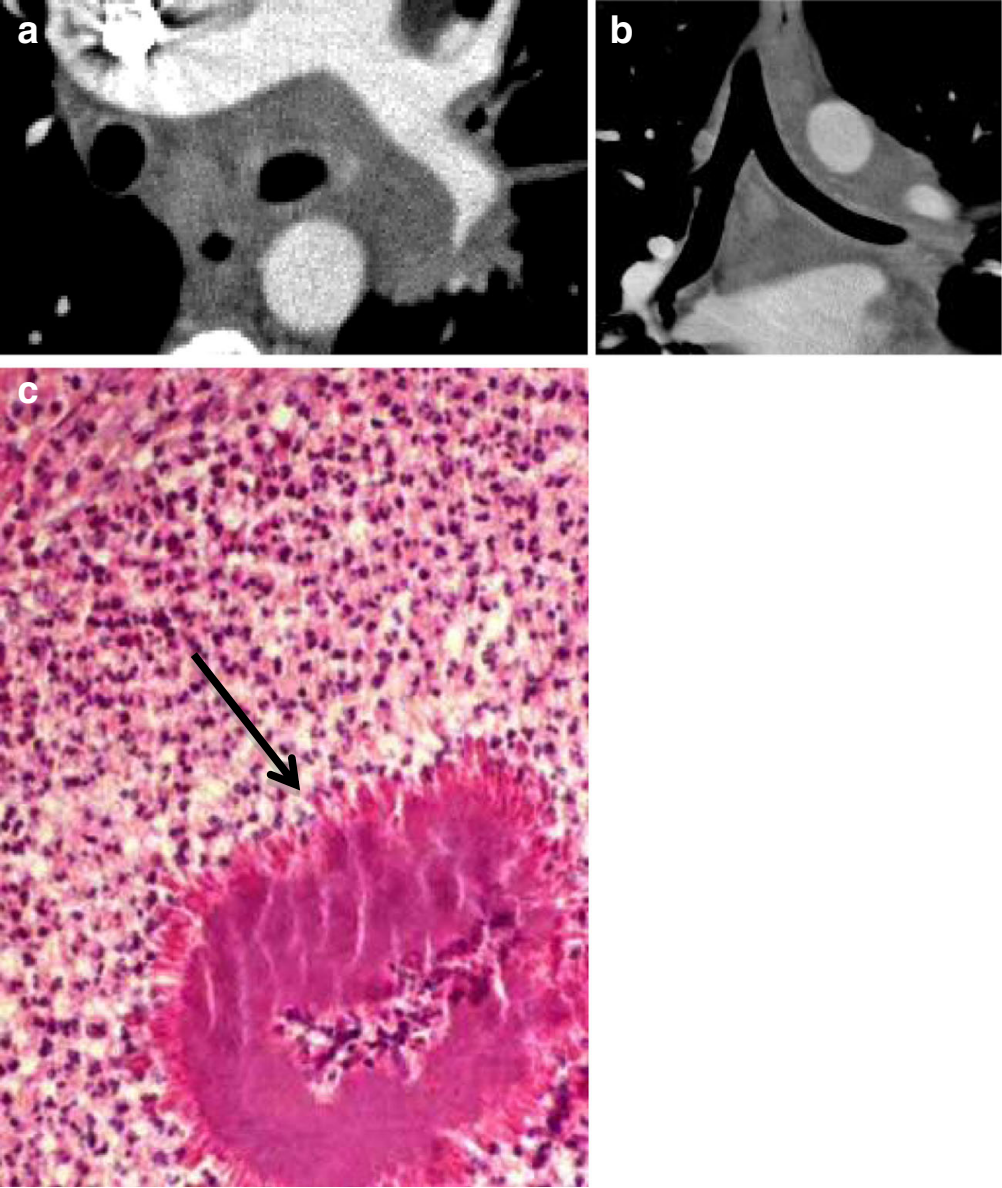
Thirty-four-year-old man with a axial and b coronal contrast enhanced CT imaging demonstrating matted mediastinal lymphadenopathy surrounding the left lower lobe bronchus with mild extrinsic narrowing and an irregular lesion of the left main bronchus. c Pathology reveals acute inflammatory exudate and *Actinomyces* colony, note characteristic sulfa granule (*arrow*)
